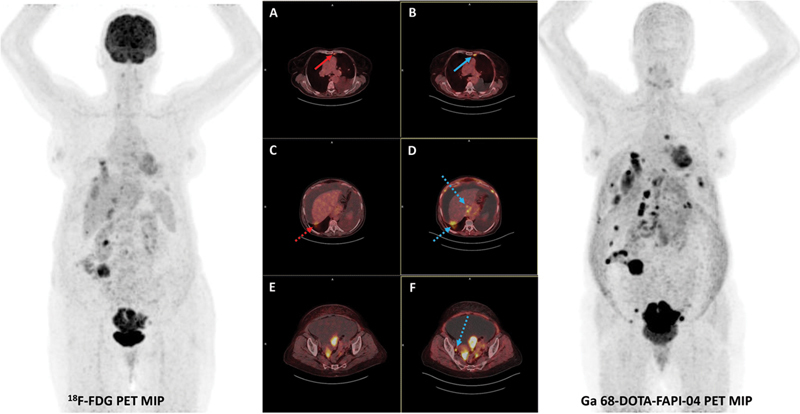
A 71-year-old woman with a metastatic lymph node located in the left parasternal region (FAPI SUVmax = 10.3, FDG SUVmax = 3.9). (
